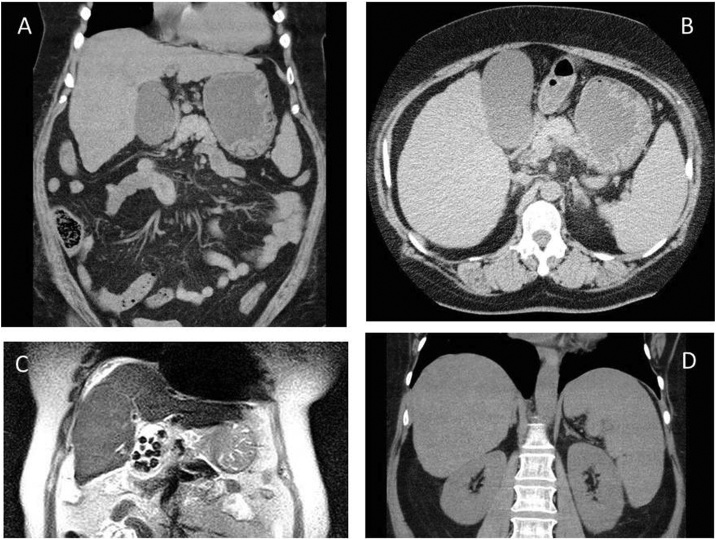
Computed tomography (CT) scan and magnetic resonance imaging findings. A. Irregular surface of the liver and dilatation of the gallbladder are seen. B. The gallbladder is medial. C. MRI revealed multiple gall stones. D. Splenomegaly is seen in the coronal plane.
